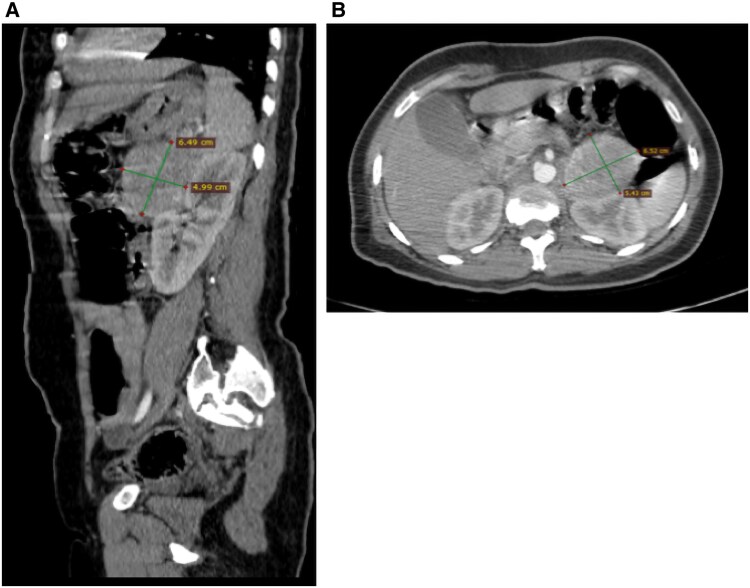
Abdominal computed tomography demonstrating a left adrenal mass. (*A*) Sagittal view. (*B*) Axial view showing a well-defined heterogeneous left adrenal tumour measuring approximately 65 × 50 × 66 mm, suggestive of pheochromocytoma.
